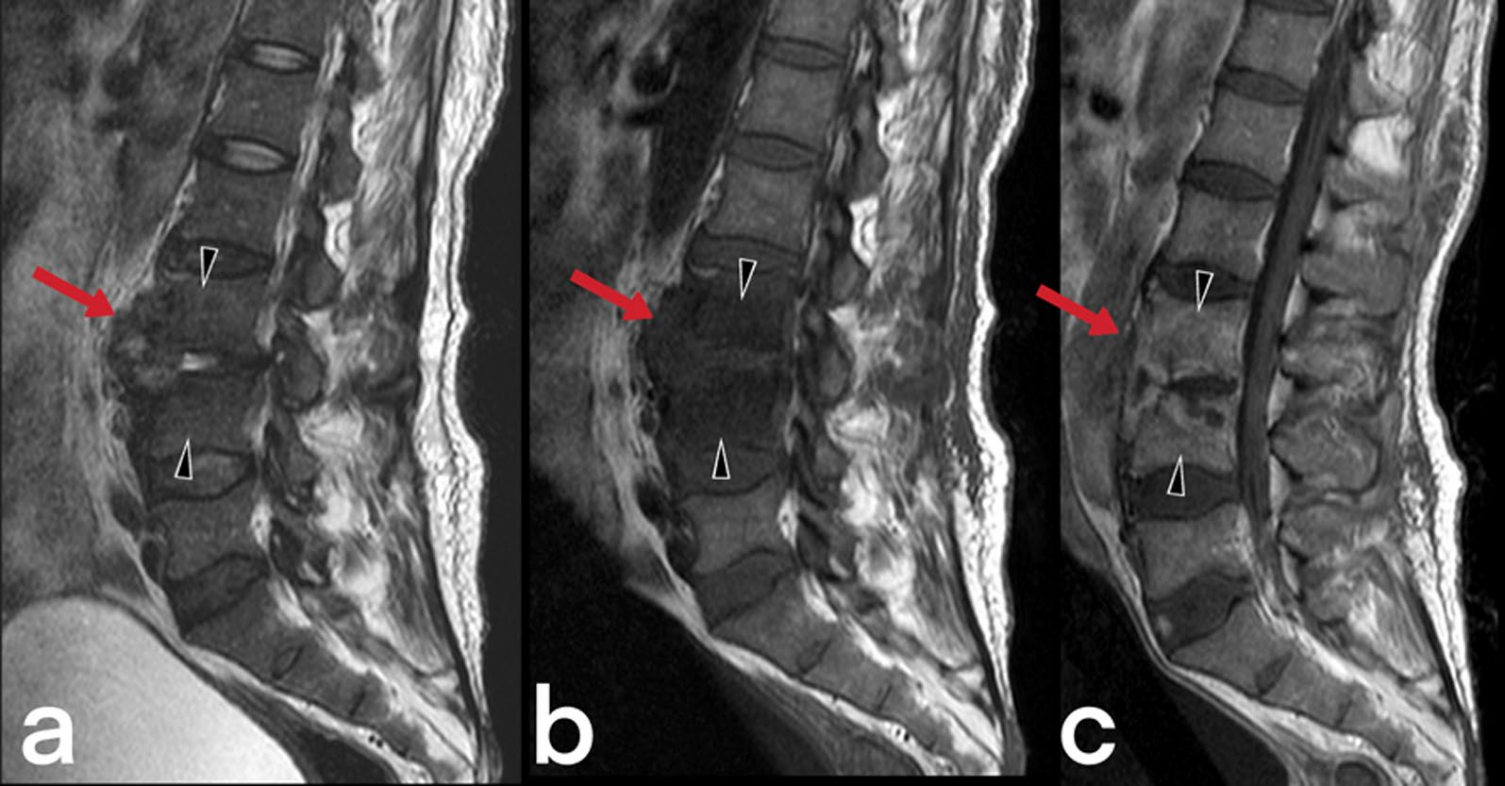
MR images of lumbar vertebrae of a 47-year-old male with tuberculous spondylodiscitis. Sagittal T2-weighted image (a) shows the heterogeneous low signal in the anterior parts of the L3 and L4 vertebral bodies and L3-4 intervertebral disc, which offers minimal anterior subligamentous extension (arrows). Pre-contrast (a) and post-contrast (b) T1 weighted images reveal diffuse contrast enhancement of both vertebral bodies in keeping with spondylodiscitis (arrowheads)
